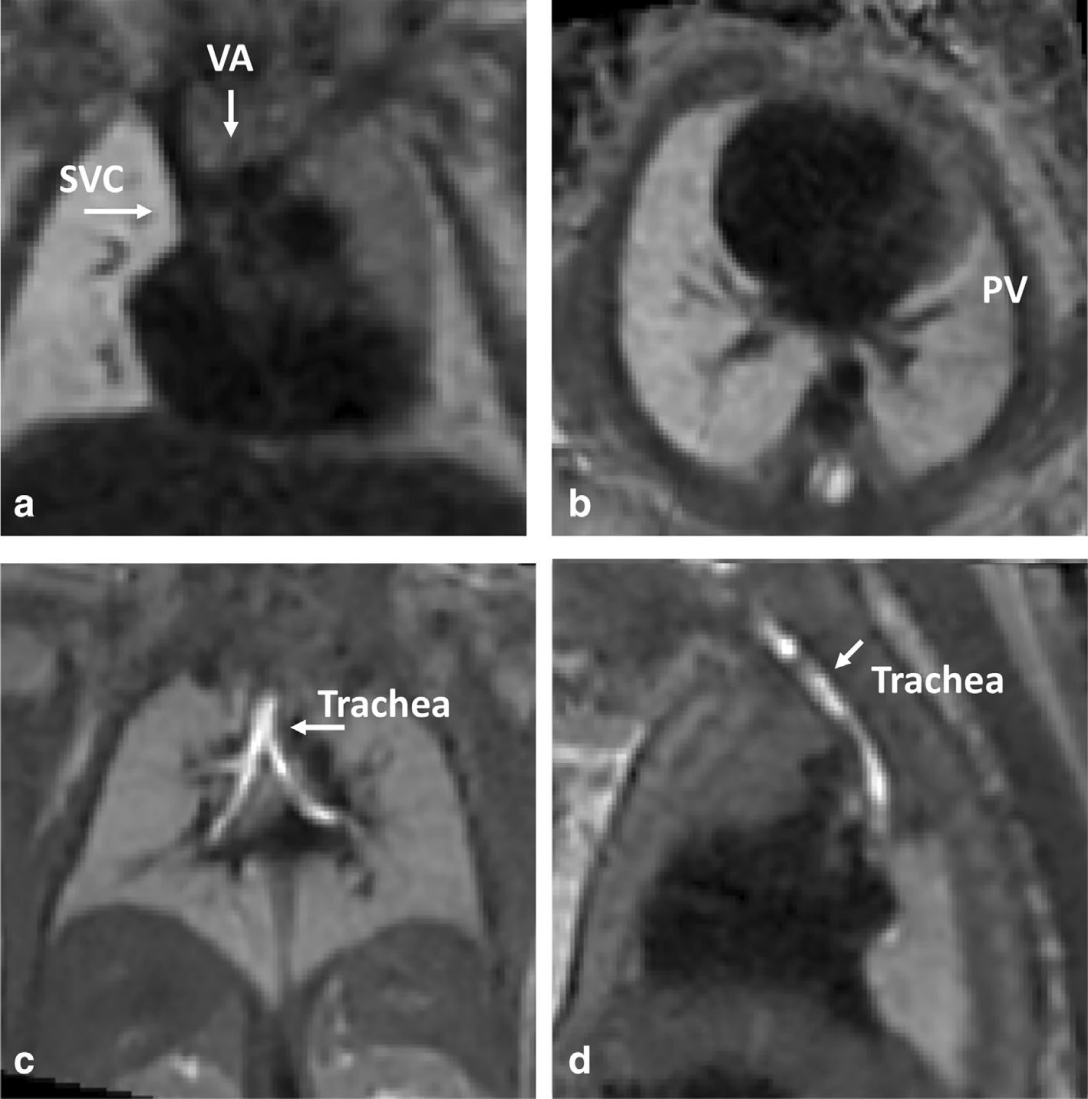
Fetal cardiovascular MRI in a fetus GA 33+ weeks allows assessment of (a) normal superior vena cava (SVC) and vena anonyma (VA) connections, and (b) normal pulmonary vein (PV) anatomy. (c, d) A fetus of GA 34+ weeks shows the development and caliber of the airways evaluated in the same 3.0 T MRI T_2_‐weighted acquisition, as well as the overall aspect of the lungs and normal position of liver (c).
